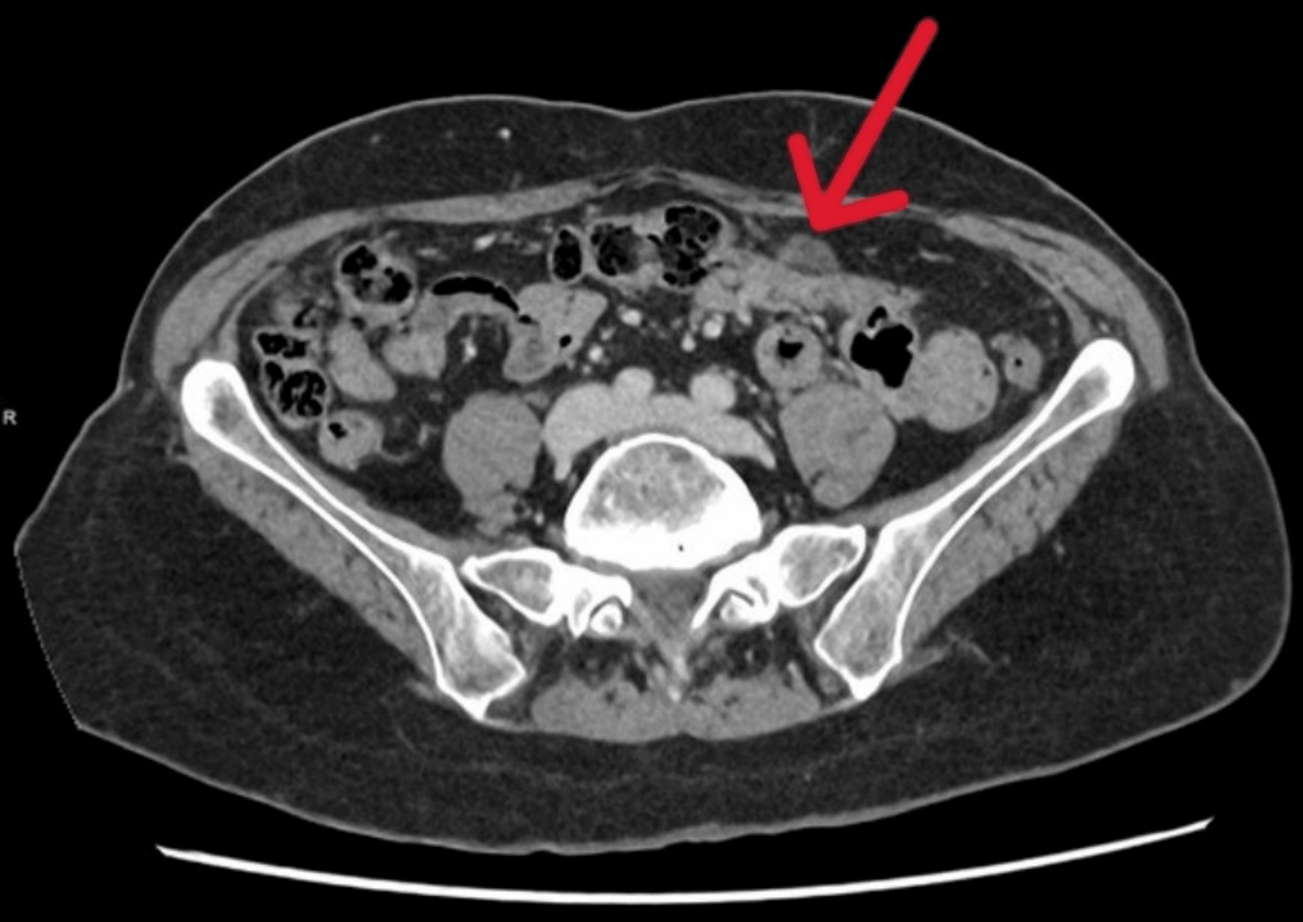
Axial CT image demonstrating cystic peritoneal lesions resembling peritoneal carcinomatosis (arrow).
